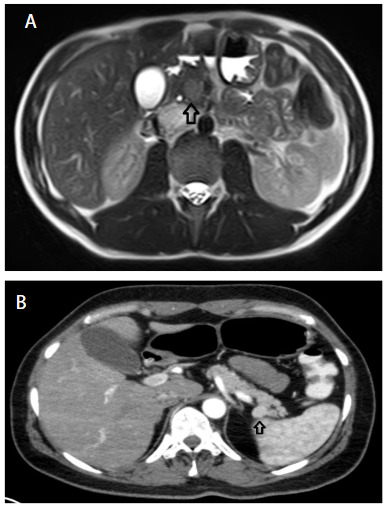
(A) MRI (T2A) images of the abdomen. Arrow shows the hyperintense insulinoma in the pancreatic head. (B) Arterial phase CT image of the abdomen. Arrow shows hypervascular, hyperdense mass lesion in the pancreatic tail.
